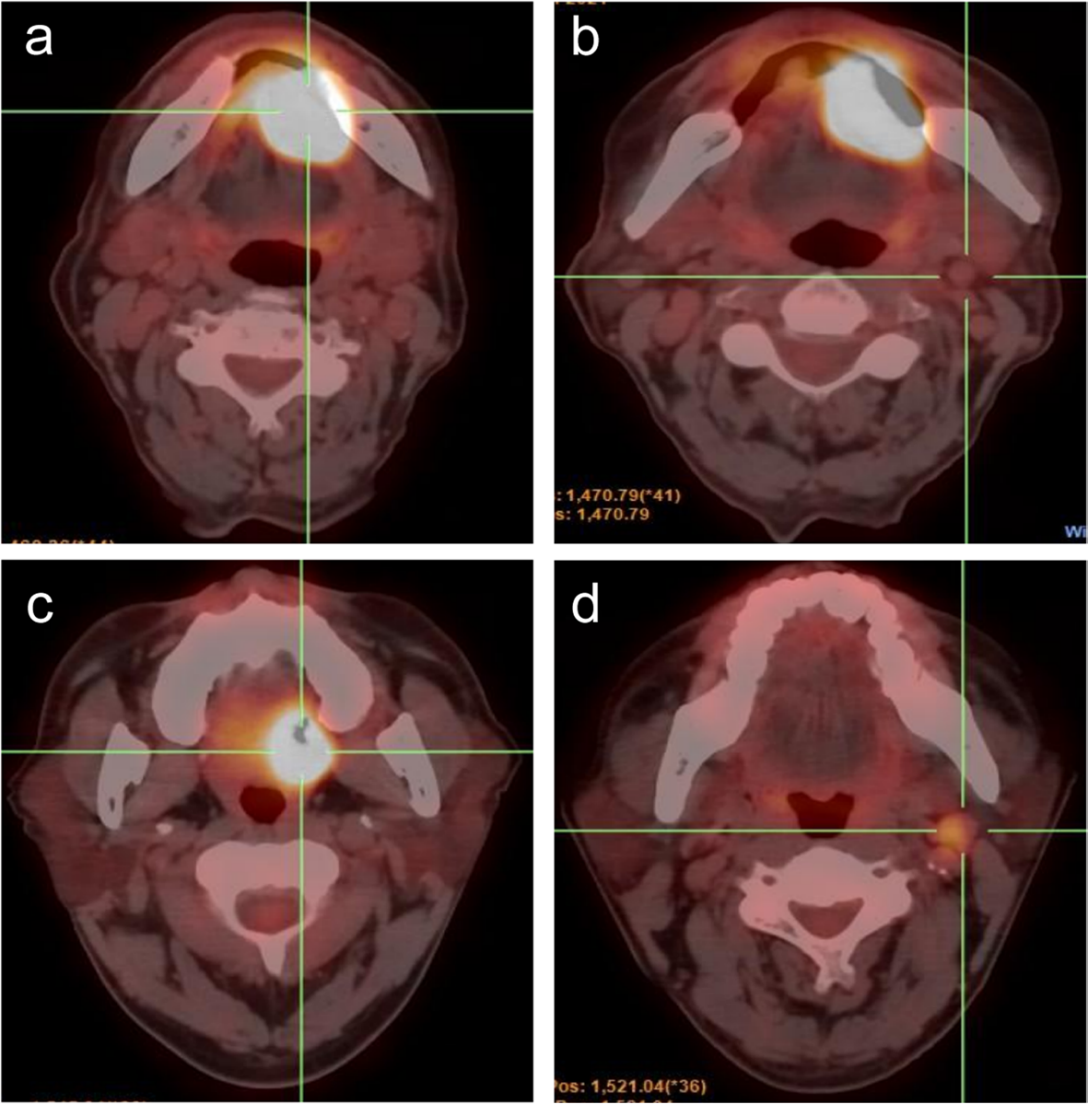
(a-b) A 63-year-old male with floor of mouth SCC. a PET/CT showed intense ^18^F-FDG uptake in the primary tumor(pathological T stage III, high probability of LN metastasis). b A LN with the most intense uptake of ^18^F-FDG was observed in the left neck side with SUVmax 1.76(Low probability of LN metastasis); The prediction model indicated the probability of nodal metastasis, which was confirmed by neck dissection (I-V). (c-d) A 55-year-old male with palate SCC. c PET/CT showed intense ^18^F-FDG uptake in the primary tumor(pathological T stage II, low probability of LN metastasis). d A LN with the most intense uptake of ^18^F-FDG was observed in the left neck side with SUVmax 4.62 (high probability of LN metastasis); The prediction model suggested probability of nodal metastasis, which was confirmed by neck dissection (I-V)
